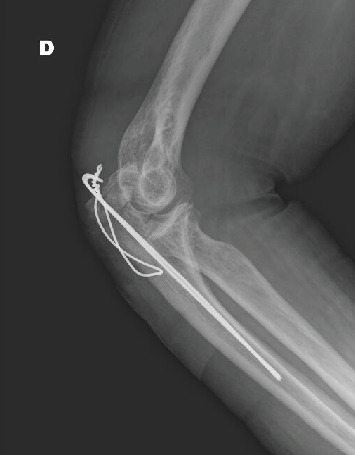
X-ray of the stabilization of the fracture of the olecranon with *Zuggurtung* technique.
